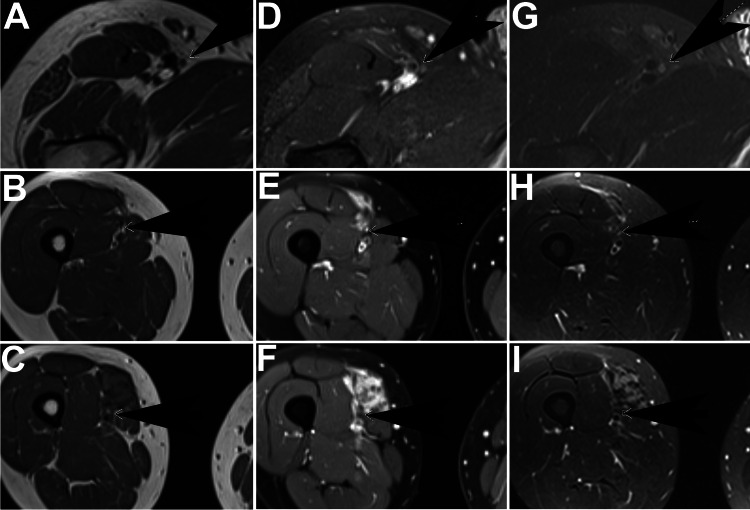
Sequential axial MR images of Case 2, continuation from Figure 4 Left column (A-C): T1 sequence; middle column (D-F): T1 fat saturation sequence post contrast; right column (G-I): T2 fat saturation. Black arrows indicate the saphenous nerve
